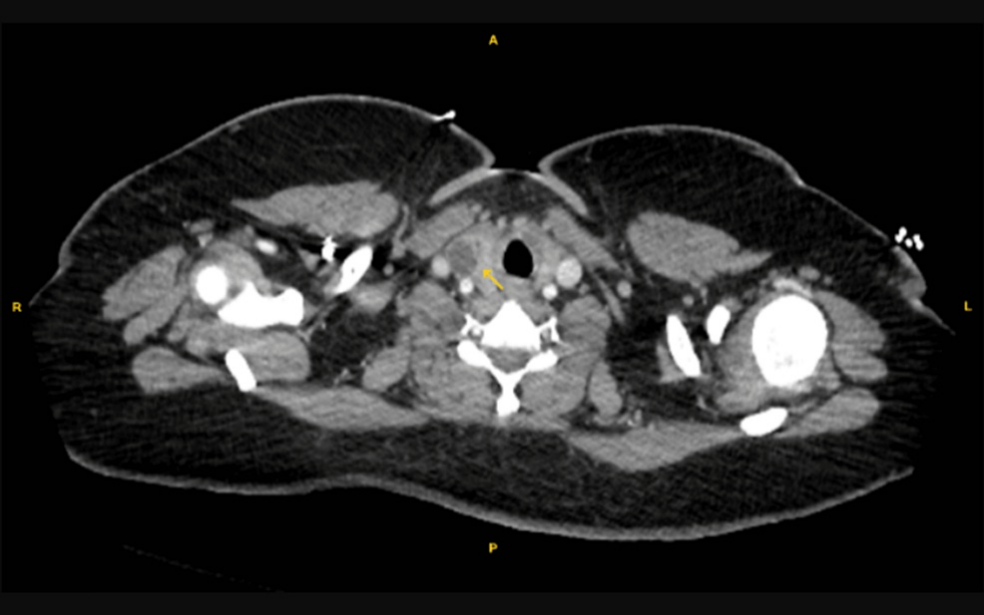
Incidental thyroid nodule noted on repeat CTA. CTA: computed tomography angiography. Yellow arrow: Thyroid nodule
